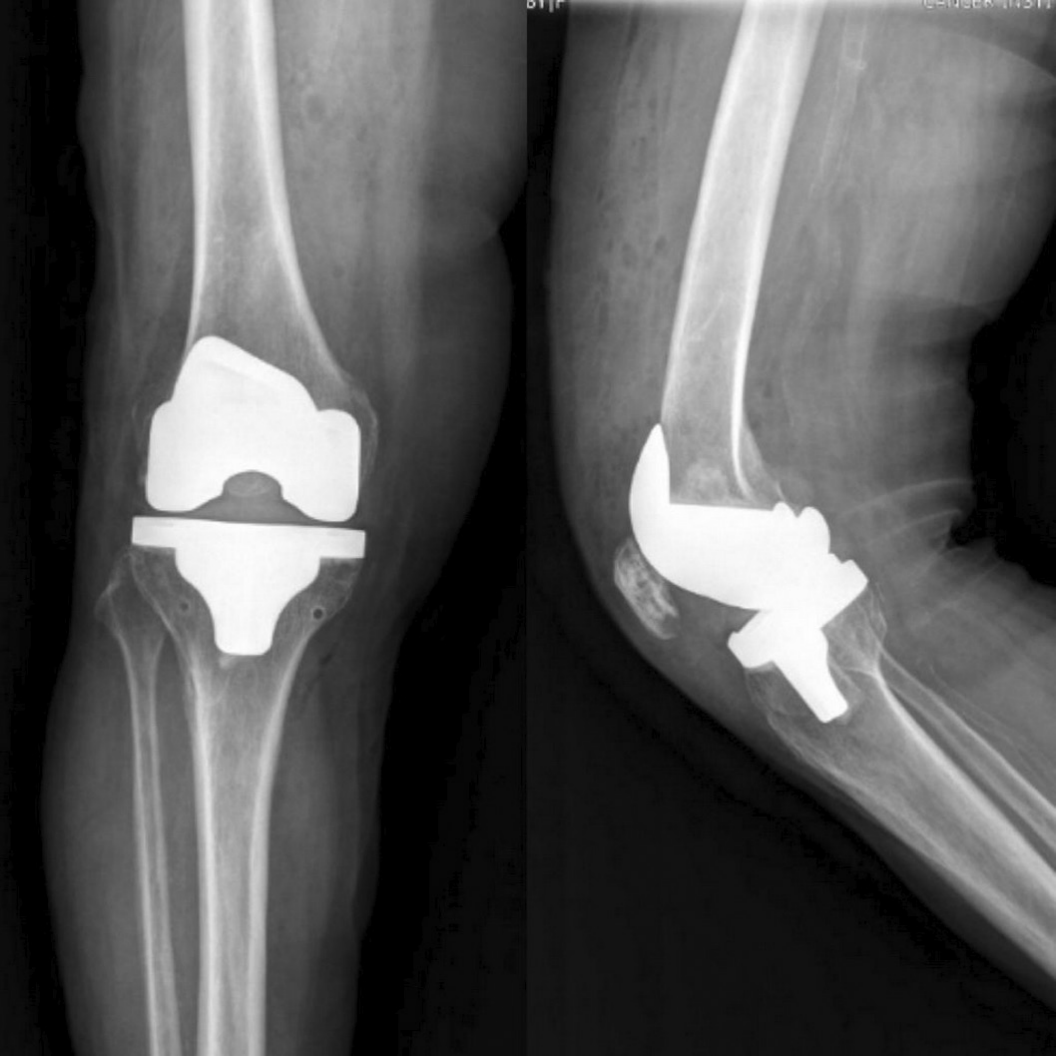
Postsurgical radiographic with acceptable alignment.
